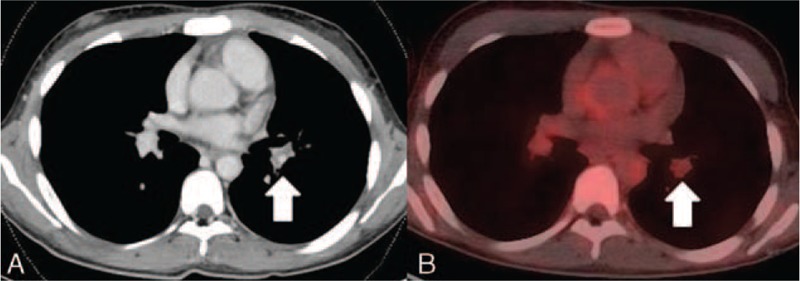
(A) Defect of contrast in the pulmonary artery without narrowing of the pulmonary artery, which suggested thrombosis (arrow). (B) PET showed no FDG accumulation in the wall of the same lesion of the pulmonary artery (arrow). FDG = 18F- fluoro-2-deoxy-D-glucose, PET = positron emission tomography.
